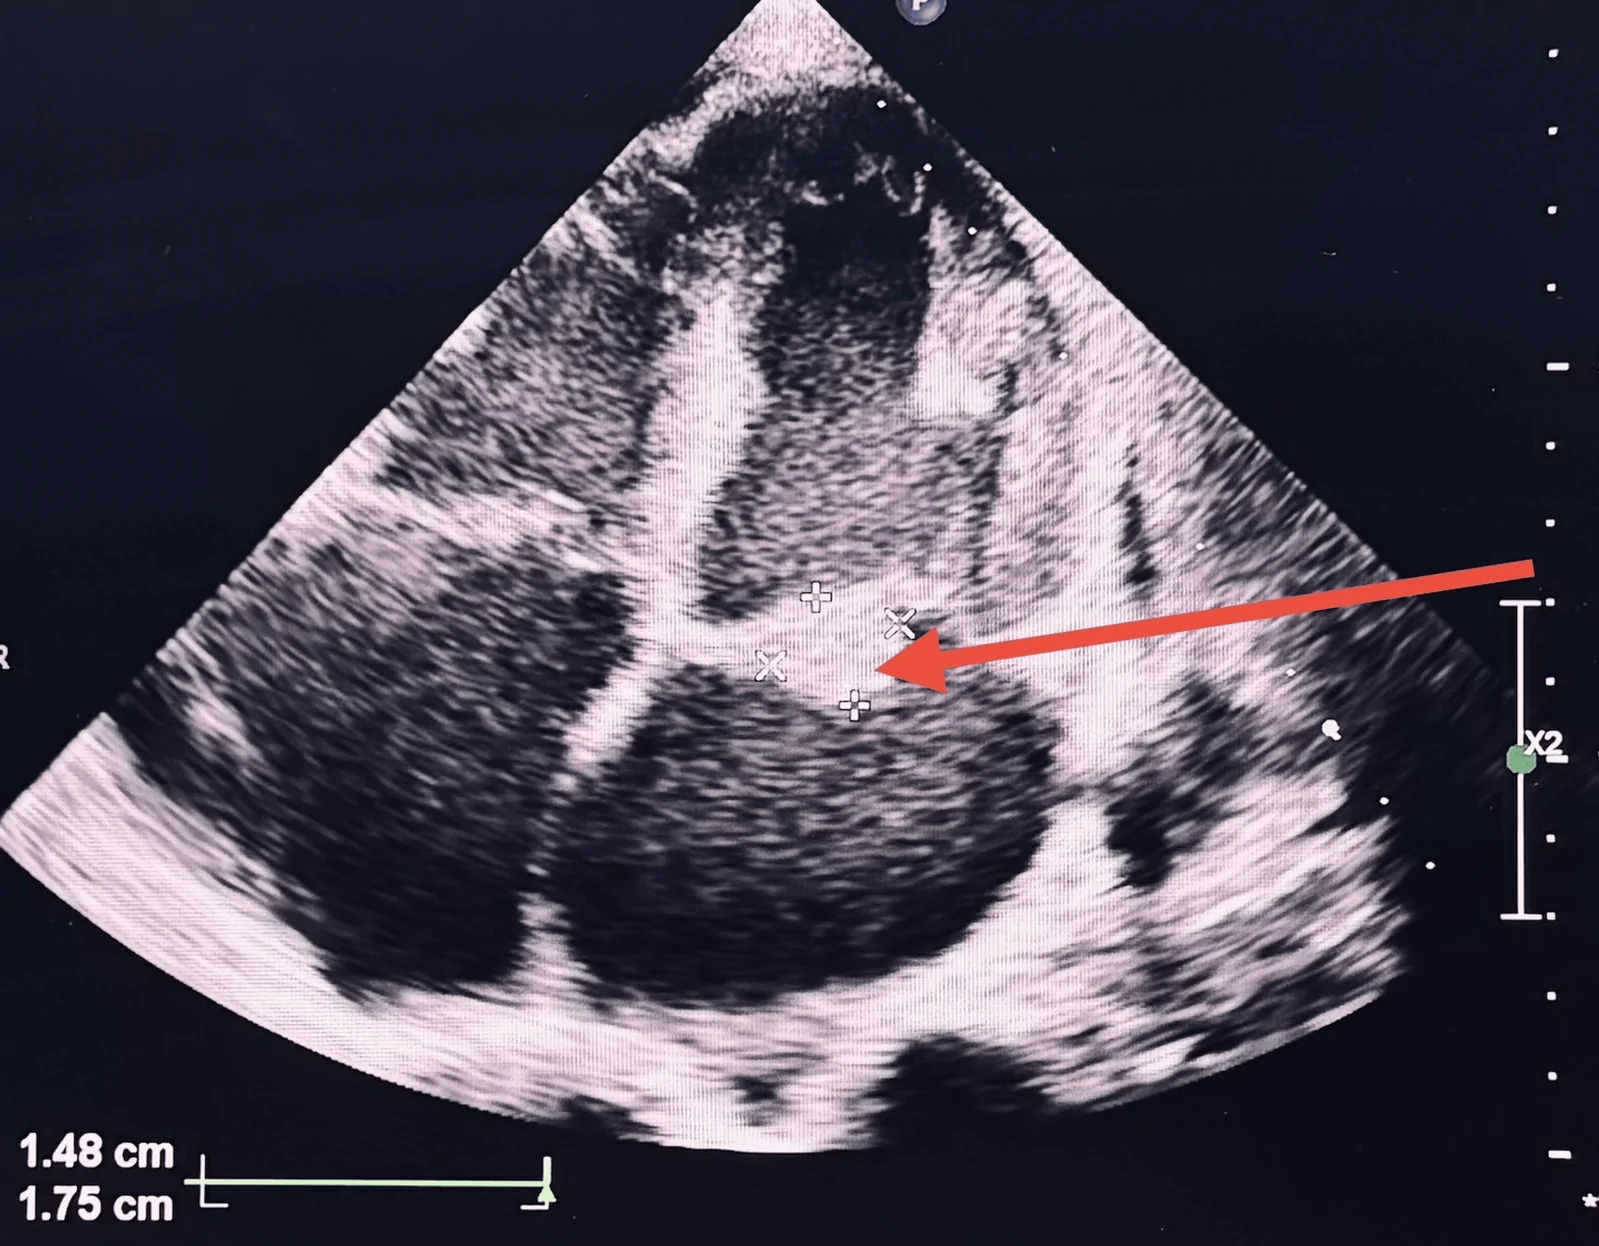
Transthoracic echocardiogram of the anterior mitral valve leaflet vegetation (red arrow) Apical four-chamber transthoracic echocardiographic image showing a pedunculated vegetation arising from the anterior mitral valve leaflet (red arrow), measuring 13 × 14 mm, suggestive of mitral valve endocarditis.
